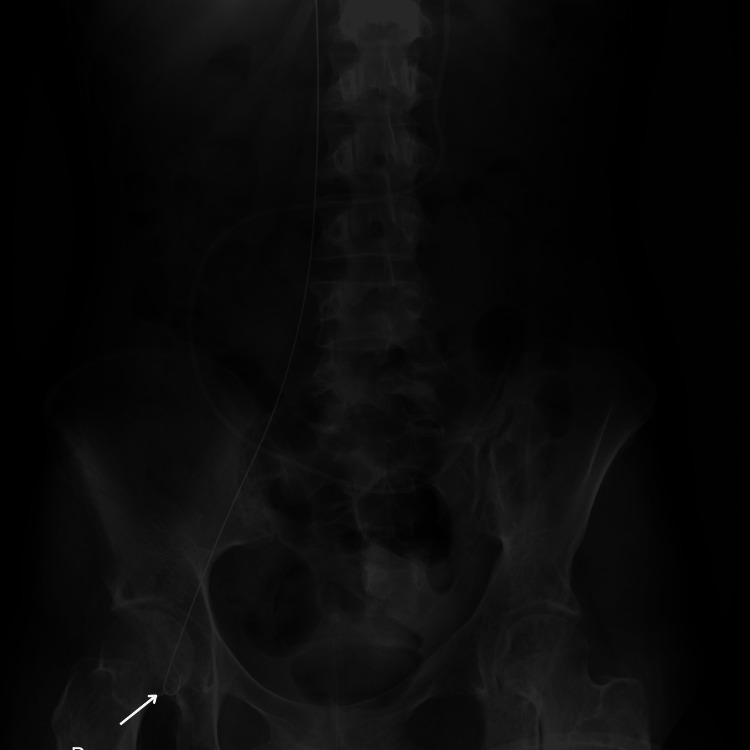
Abdominal X-ray The distal part of the guidewire
